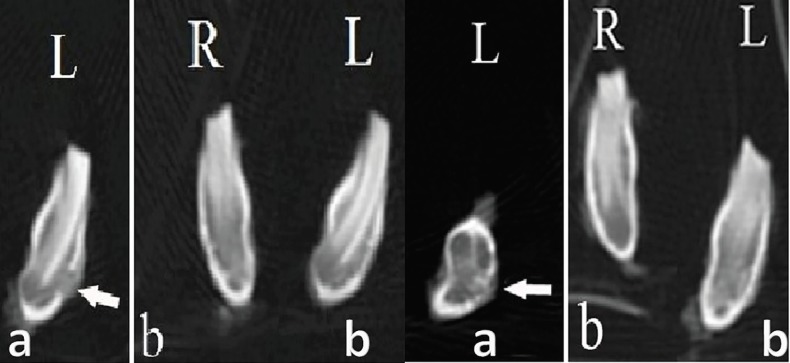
Coronal CT scanning observation after 4 weeks. Left aL: the control defect, Left bR: the defect filled with fibrin glue; Left bL: the defect filled with autologous bone graft. Coronal CT scanning observation 8 weeks after surgery. Right aL: the control defect, Right bR: the defect filled with fibrin glue; Right bL: the defect filled with autologous bone graft.
